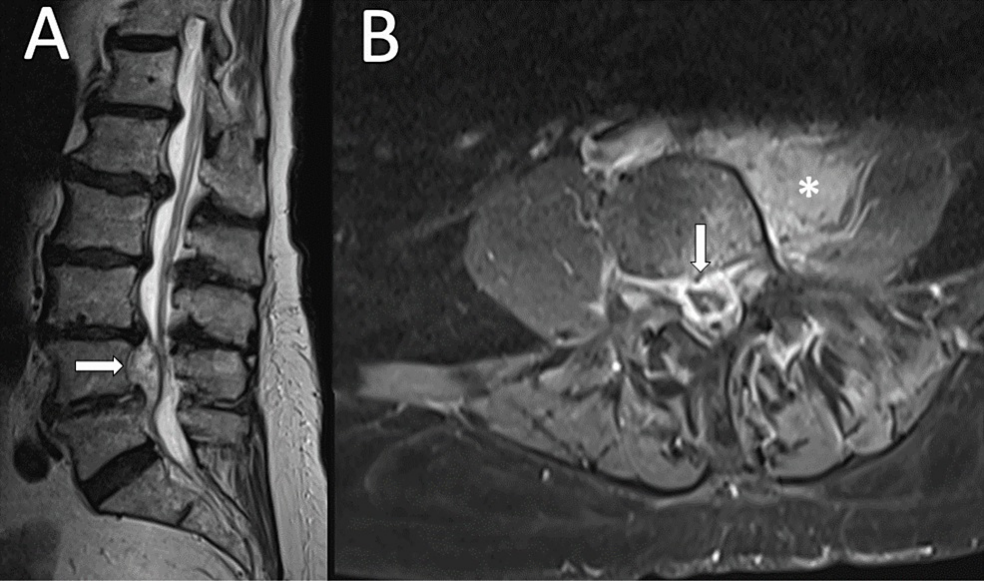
MRI revealed an epidural abscess A: T2 imaging displaying the epidural abscess. B: Axial T1 with contrast evidencing the epidural component (arrow) and the psoas abscess (*).
